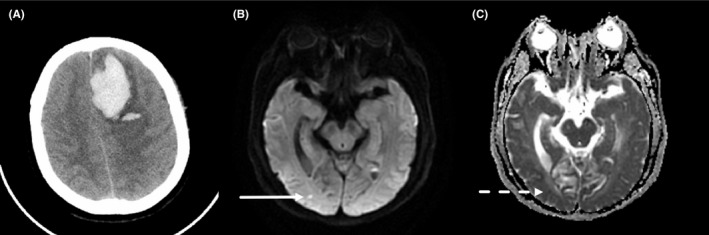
In a 43‐y‐old man with left frontal lobe hemorrhage (A), diffusion‐weighted imaging (DWI) shows a small remote ischemic lesion on the right occipital lobe (B), with corresponding low signal intensity in apparent diffusion coefficient (ADC) map (C)
